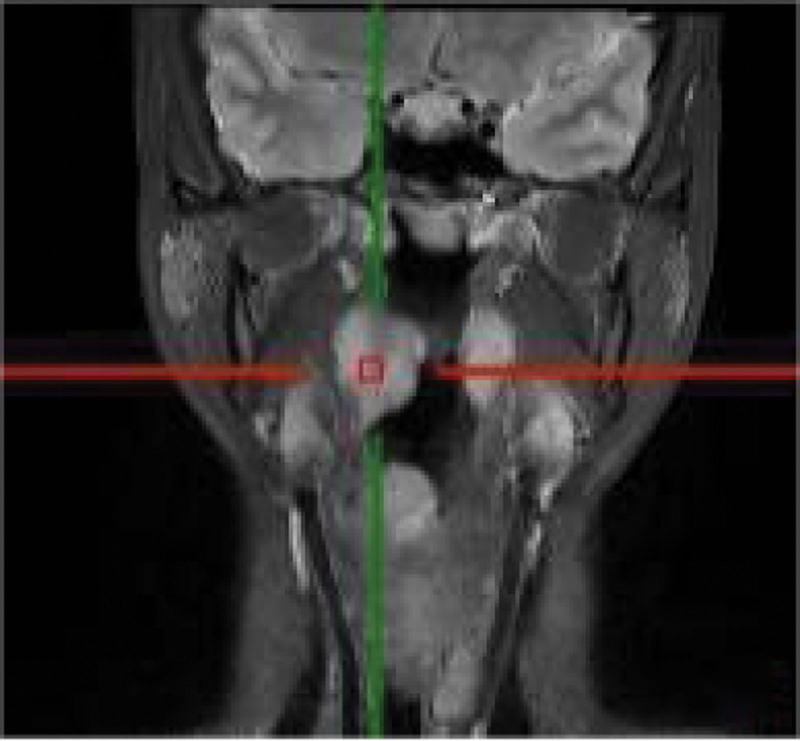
Head and Neck MRI of the coronal section.
